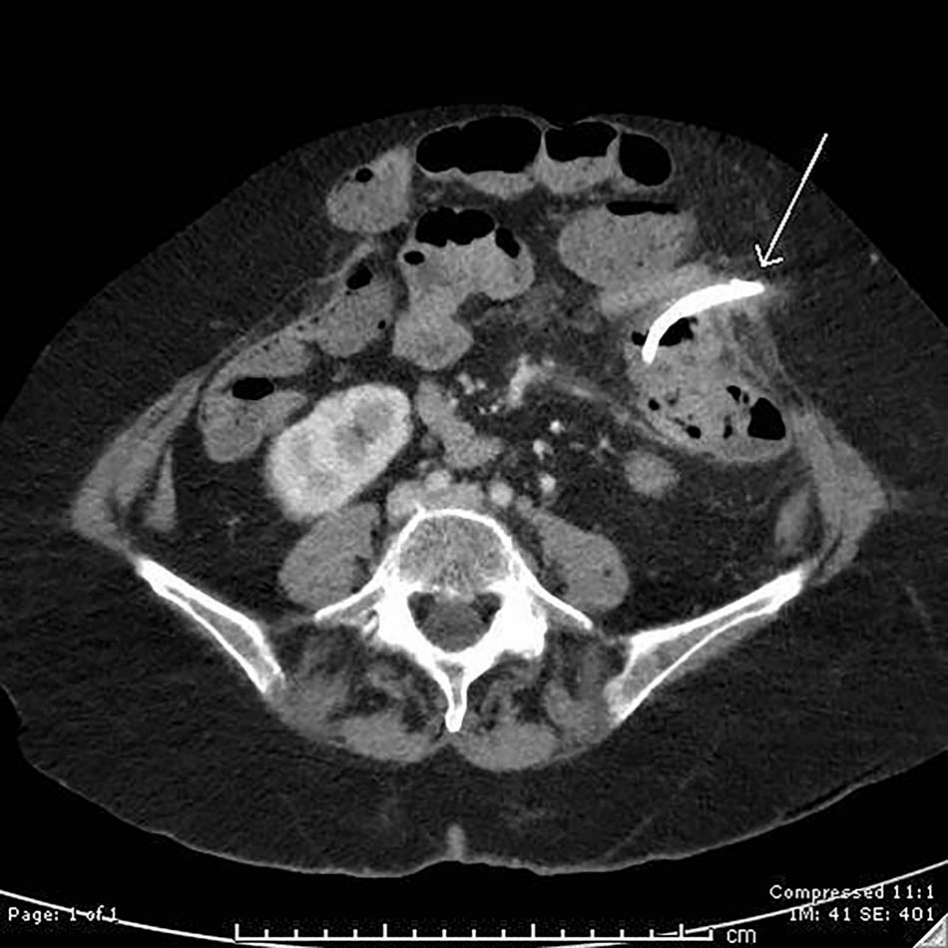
Axial computed tomography (CT) image demonstrating perforation of a migrated biliary stent into the anterior abdominal wall.
